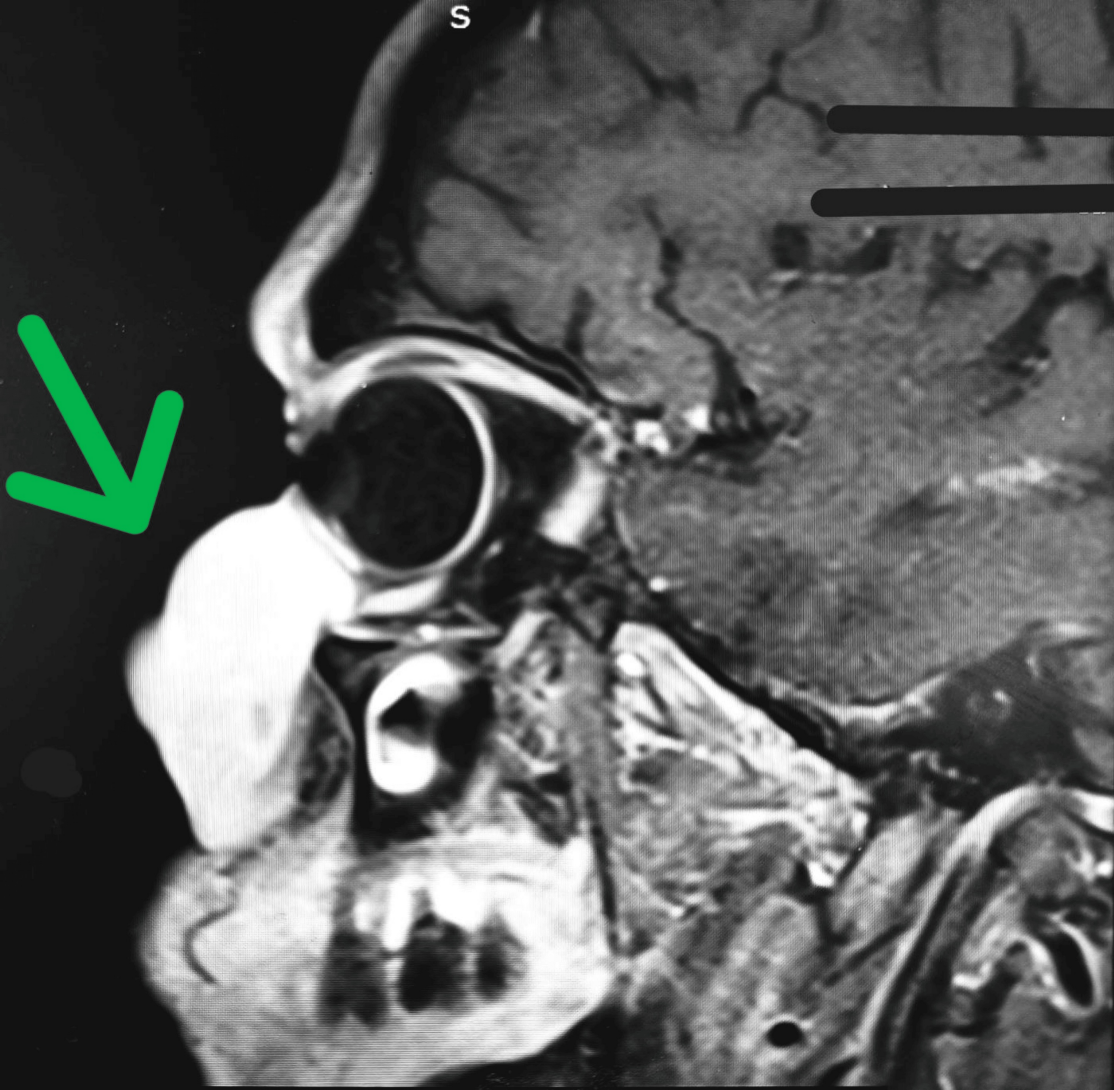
Sagittal view of MRI of the lesion indicated by the green arrow
